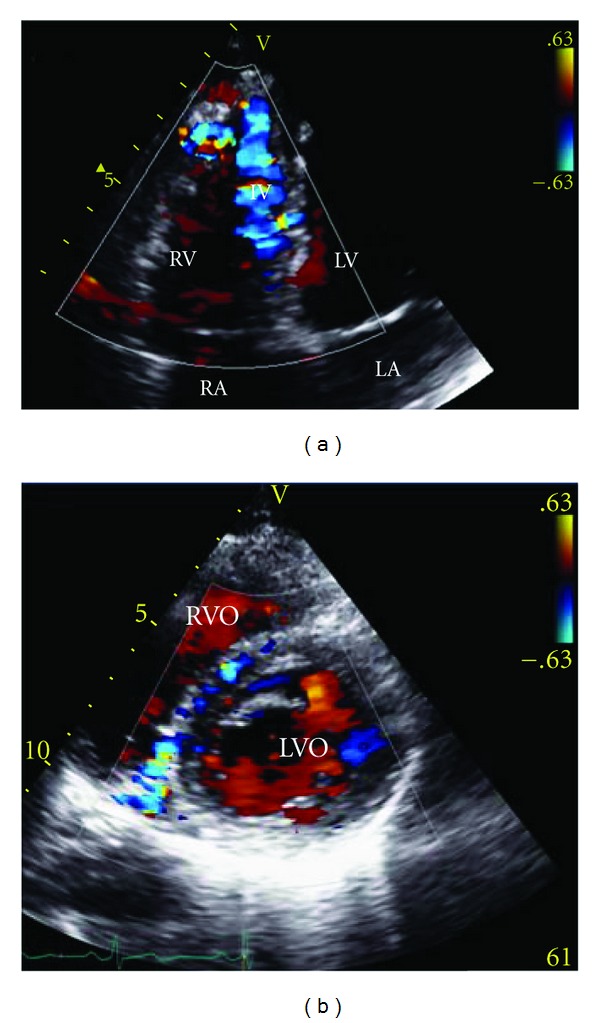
Transthoracic colour-flow Doppler echocardiography. (a) Four-chamber view. (b) Parasternal short axis view. Both images show multiple collateral vessels within the IV septum and abnormal signals in the coronary arteries, indicating dilatation and tortuosity. RA: right atrium; LA: left atrium; LV: left ventricle; RV: right ventricle; RVO: right ventricle outflow; LVO: left ventricle outflow; IV: interventricular.
